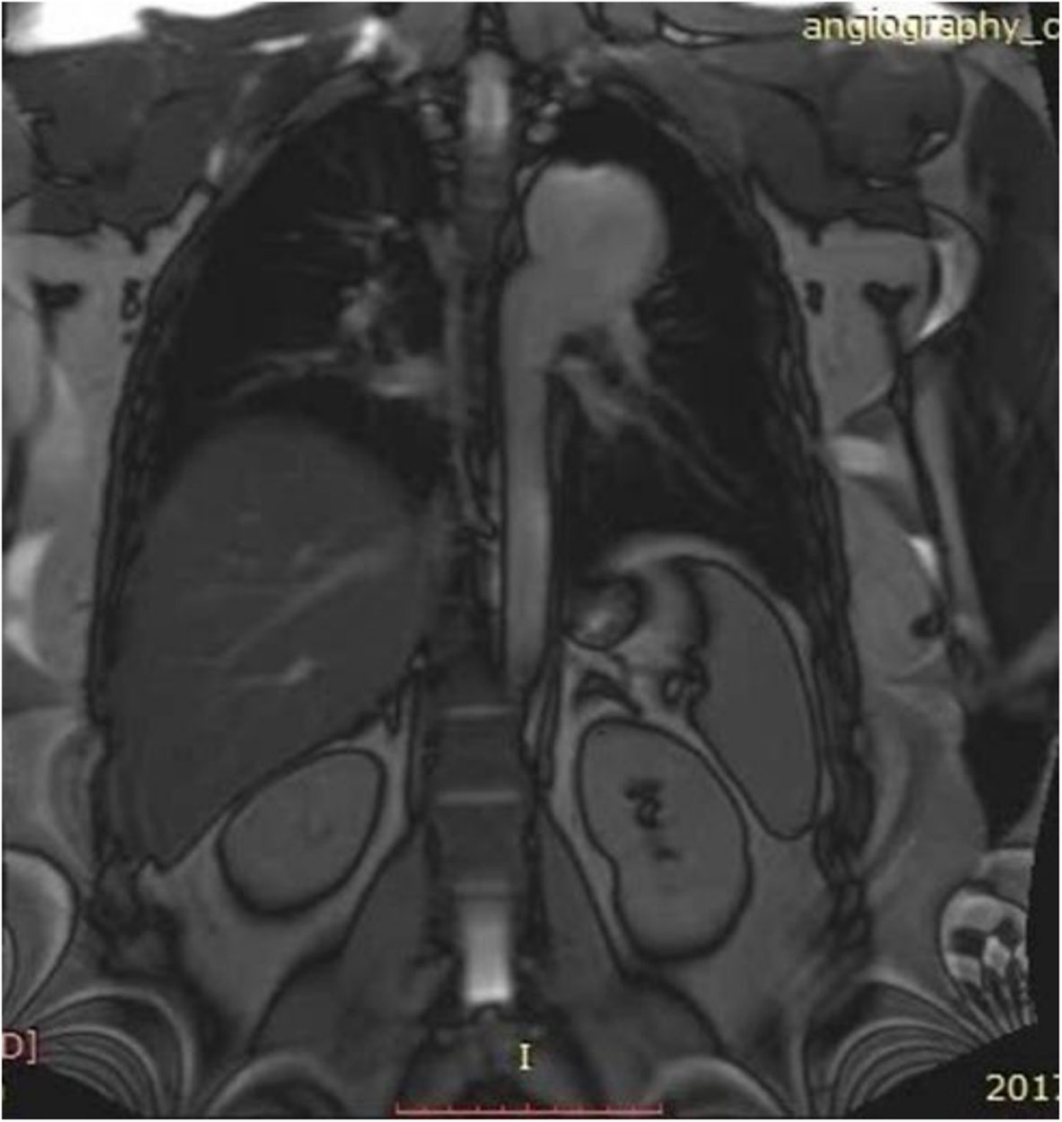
Cardiac magnetic resonance images showing aneurysmal dilation in distal part of aortic arch and proximal part of descending aorta (maximum diameter of 50 mm)
